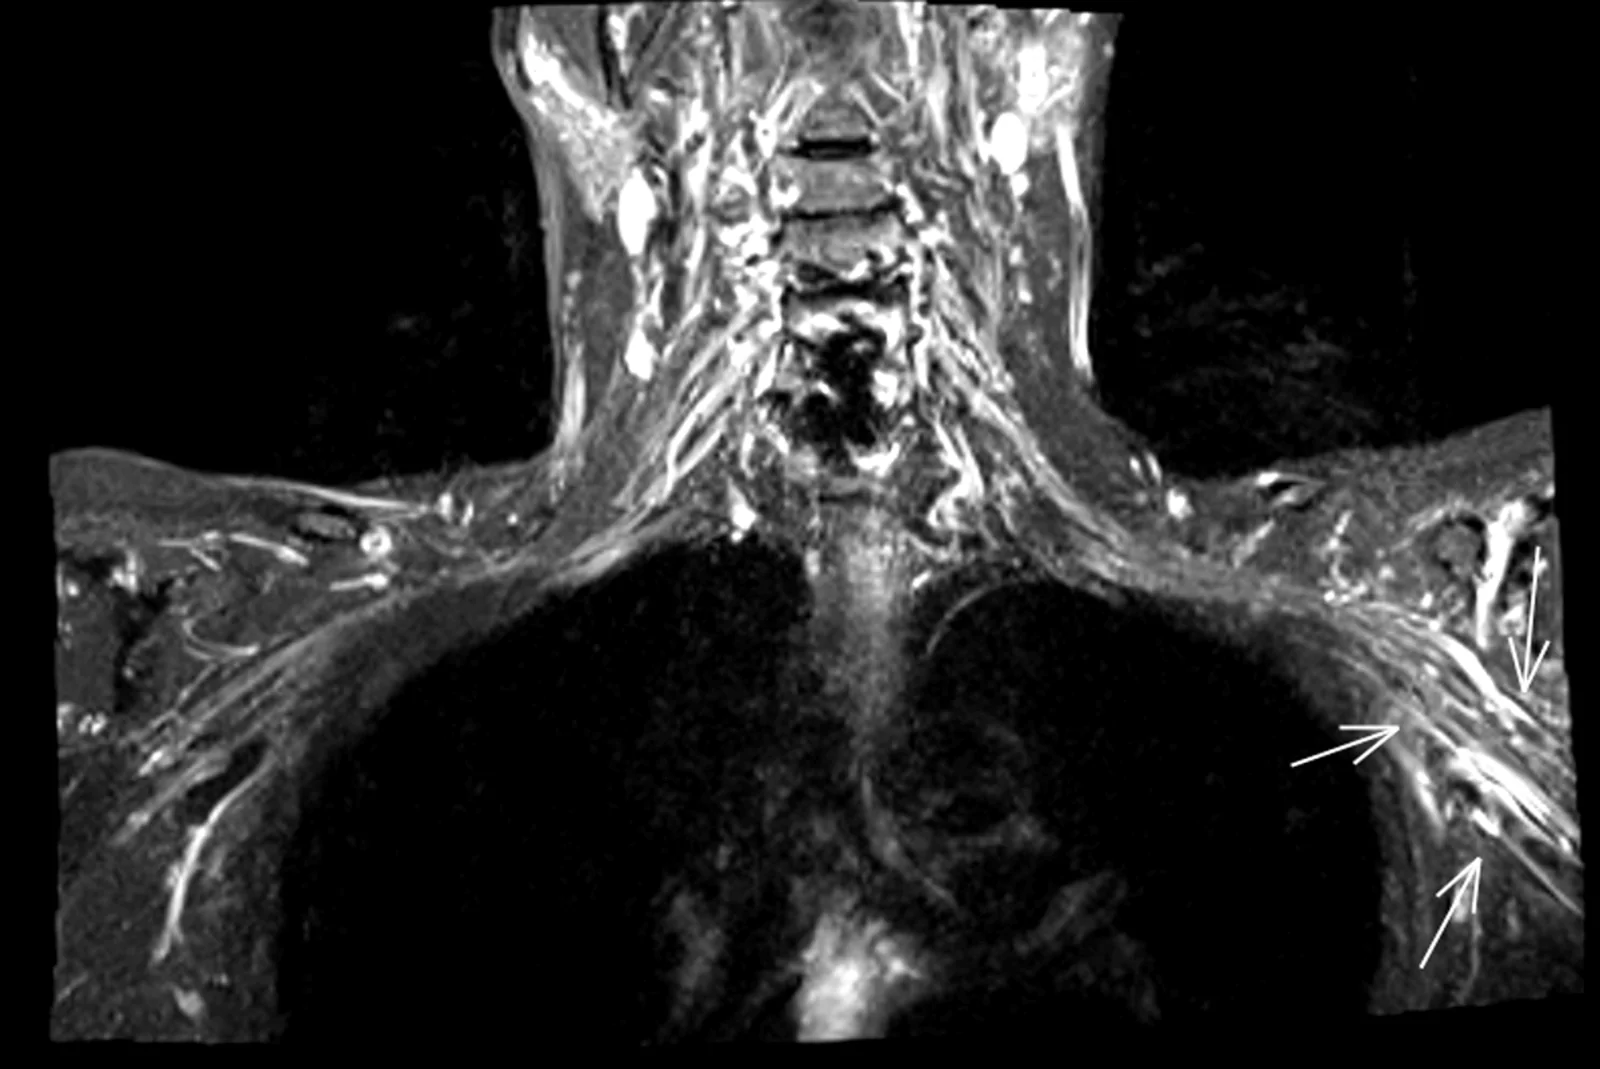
Coronal section of a brachial plexus MRI. The arrows show an increased signal from the proximal nerve tracts.
